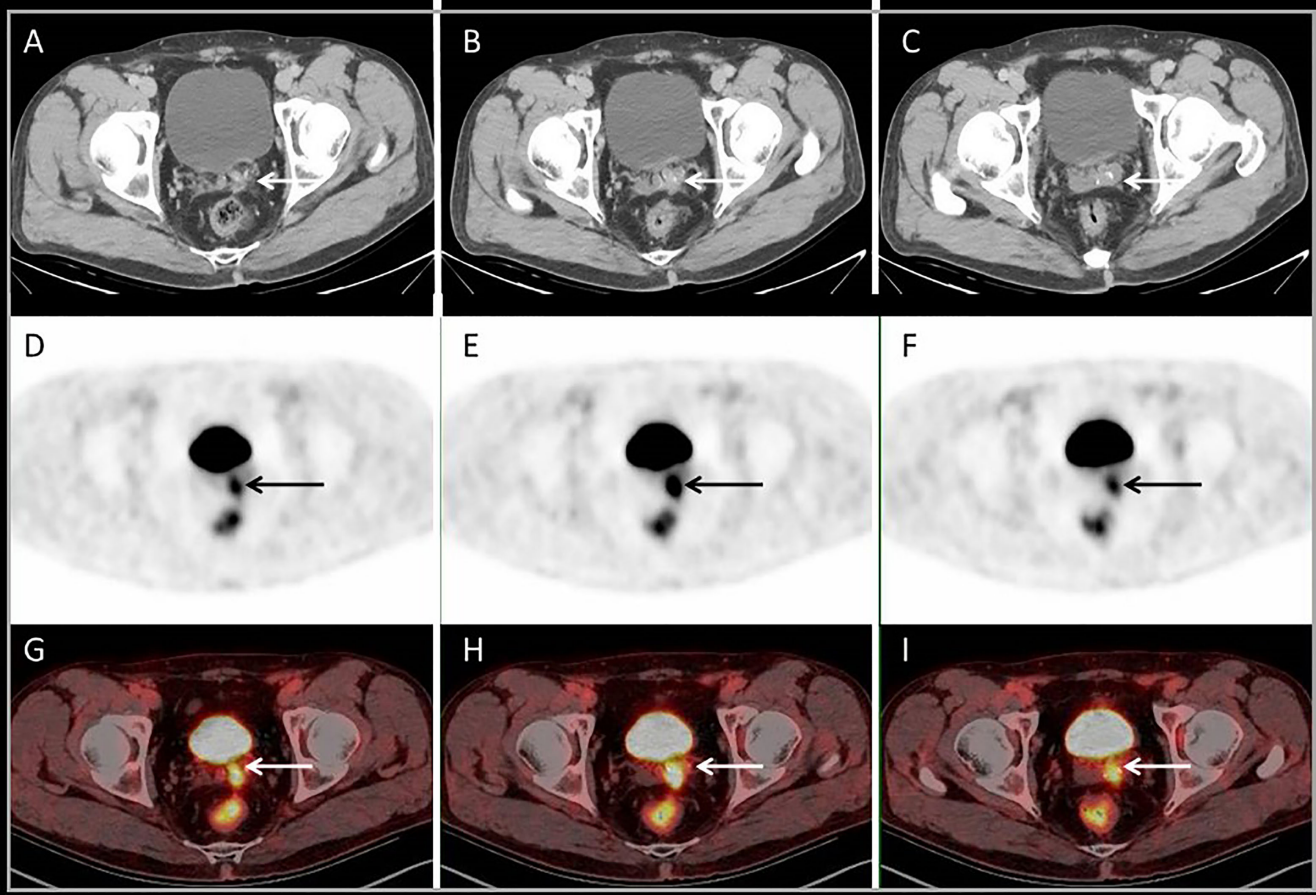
Images of a 52-year-old male with single liver metastasis after surgery of sigmoid cancer resection. Conventional abdomen CT showed no EHD (A–C). PET and PET/CT fusion images showed a peritoneal nodule adjacent to the left seminal vesicle gland (D–I). The surgery extent changed from liver only to combining live and peritoneal nodule (minor impact).
